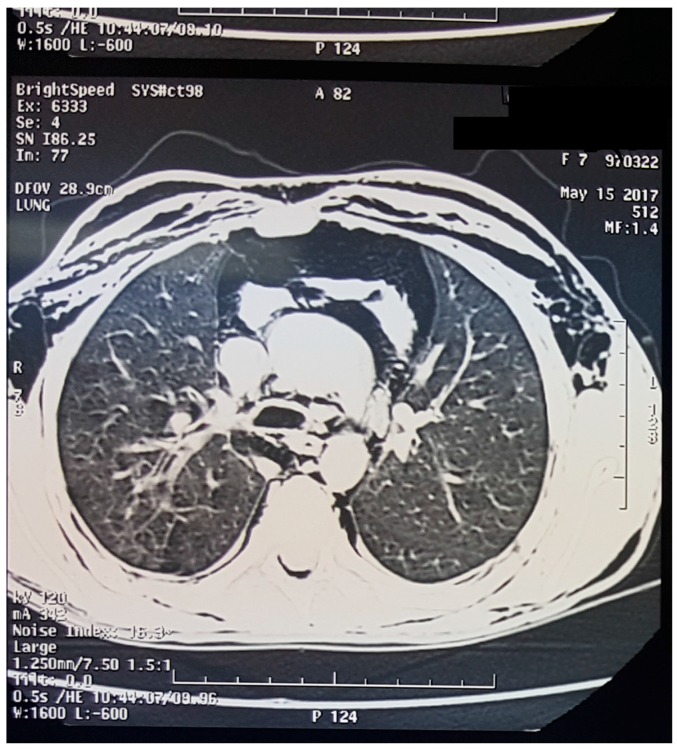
Computed tomography (CT) scan showing pneumomediastinum, pneumopericardium and emphysema.
